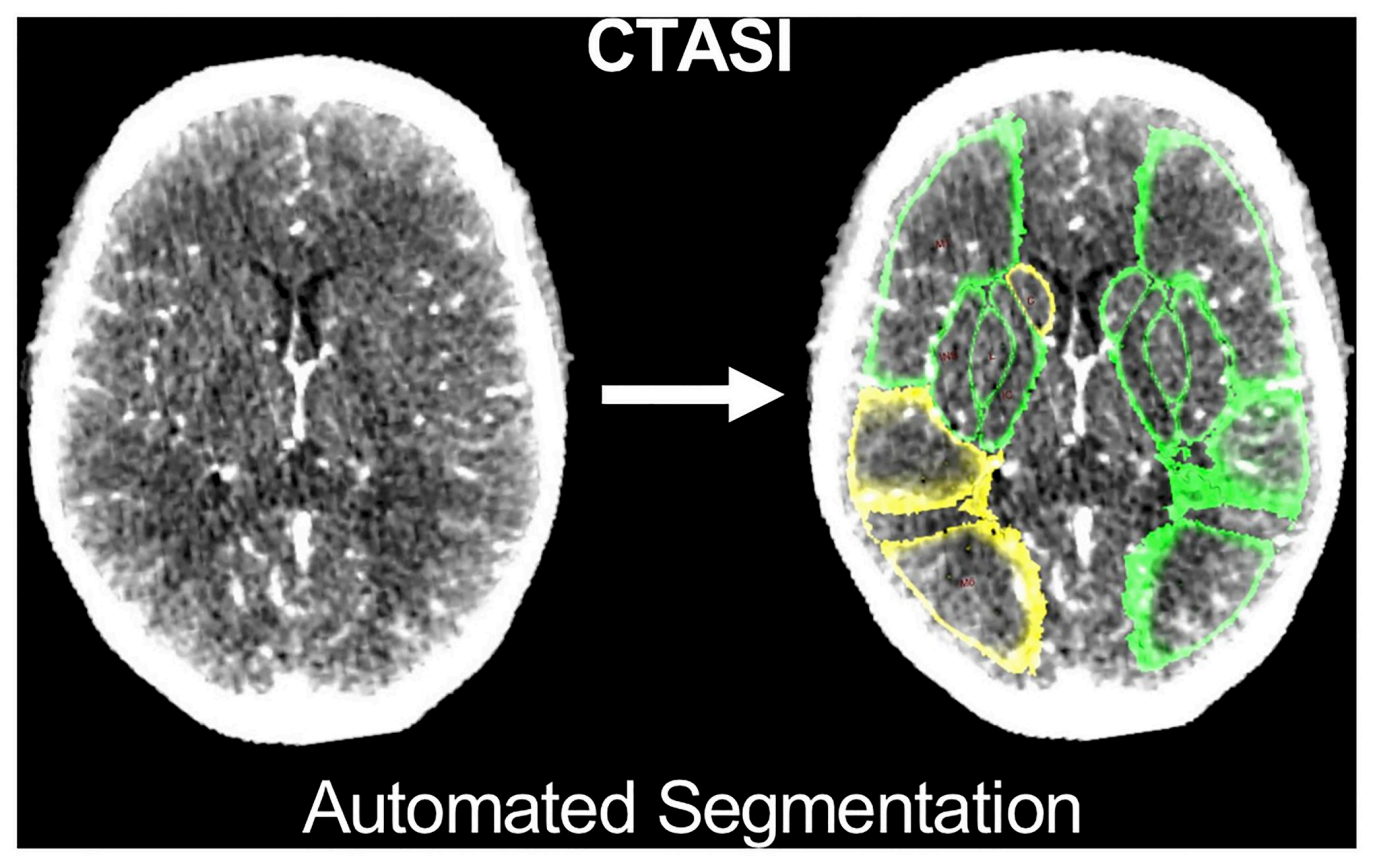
Segmentation of ASPECTS regions on CTASI. Abbreviations: ASPECTS, Alberta Stroke Program Early CT Score; CTASI, CT angiography source images; C, Caudate Nucleus; L, Lentiform Nucleus; C, Caudate; M1, M2 cortical regions of the ASPECTS. Colors indicate reduction in regional x-ray attenuation compared with the contralateral hemisphere (green = difference < 1 HU; yellow = difference ≥ 1 and < 3 HU; red = difference > 3 HU).
